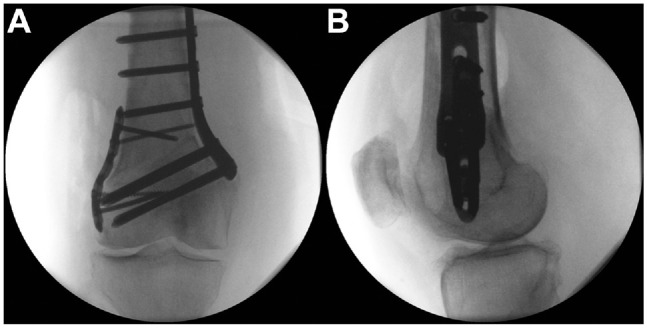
Fixation of the lateral hinge of a dislocated hinge fracture using a preshaped 5-hole, 3.5-mm locking compression plate. (A) Anteroposterior and (B) lateral radiographs.
